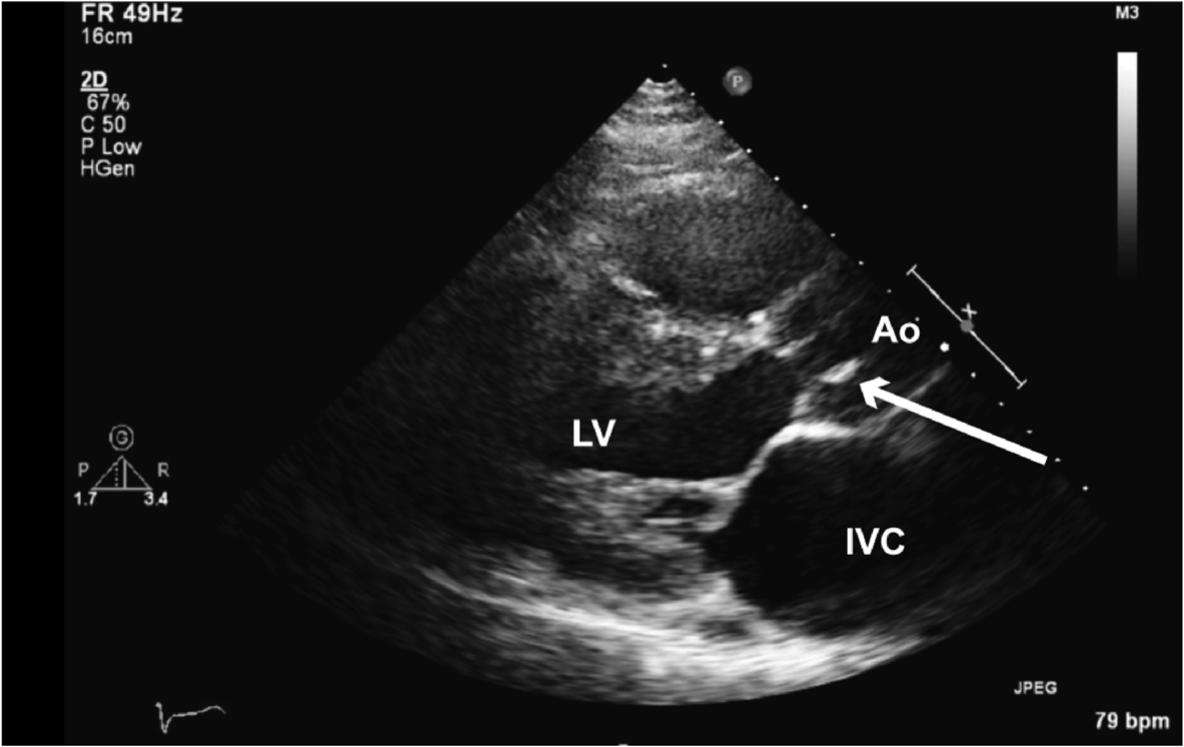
Echocardiogram showing mobile aortic valve vegetation
